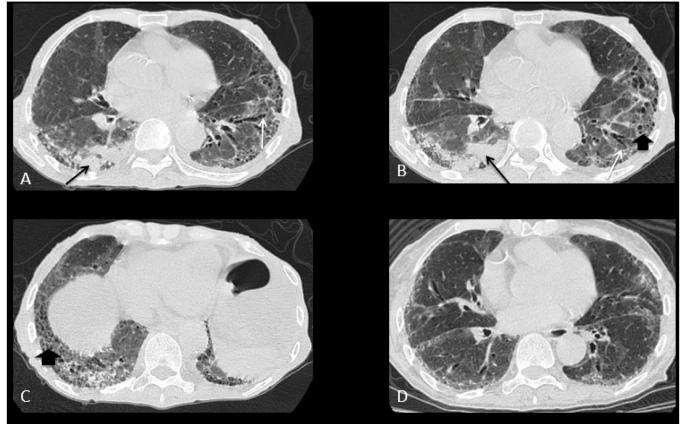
(A) and (B): Chest CT, pulmonary window, axial: right lower lobe consolidation (black arrows); (A), (B), and (C): Findings consistent with UIP: predominantly peripheral and basal interlobular septal thickening, reticulations, traction bronchiectasis (white arrows), and honeycombing (thick arrow); (D): CT performed eight months prior, without consolidation.
